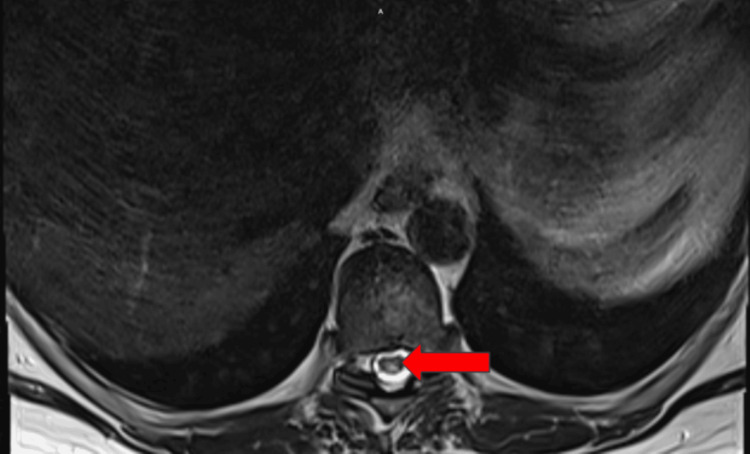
Axial view of cord signal abnormalities confined to the dorsal columns. The red arrow indicates the areas of subacute combined degeneration on MRI.
